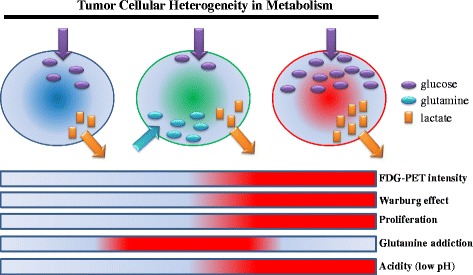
Tumor heterogeneity in metabolism. The degree of addiction to glucose or glutamate differs among various types of cancer cells. Tumor cells robustly importing glucose via the GLUT1 transporter are responsible for the high intensity of FDG-PET in the clinical settings. Cancer cells that express high levels of GLUT1 also induce a low-pH acidic tumor microenvironment, thereby increasing the invasive potential of tumors
